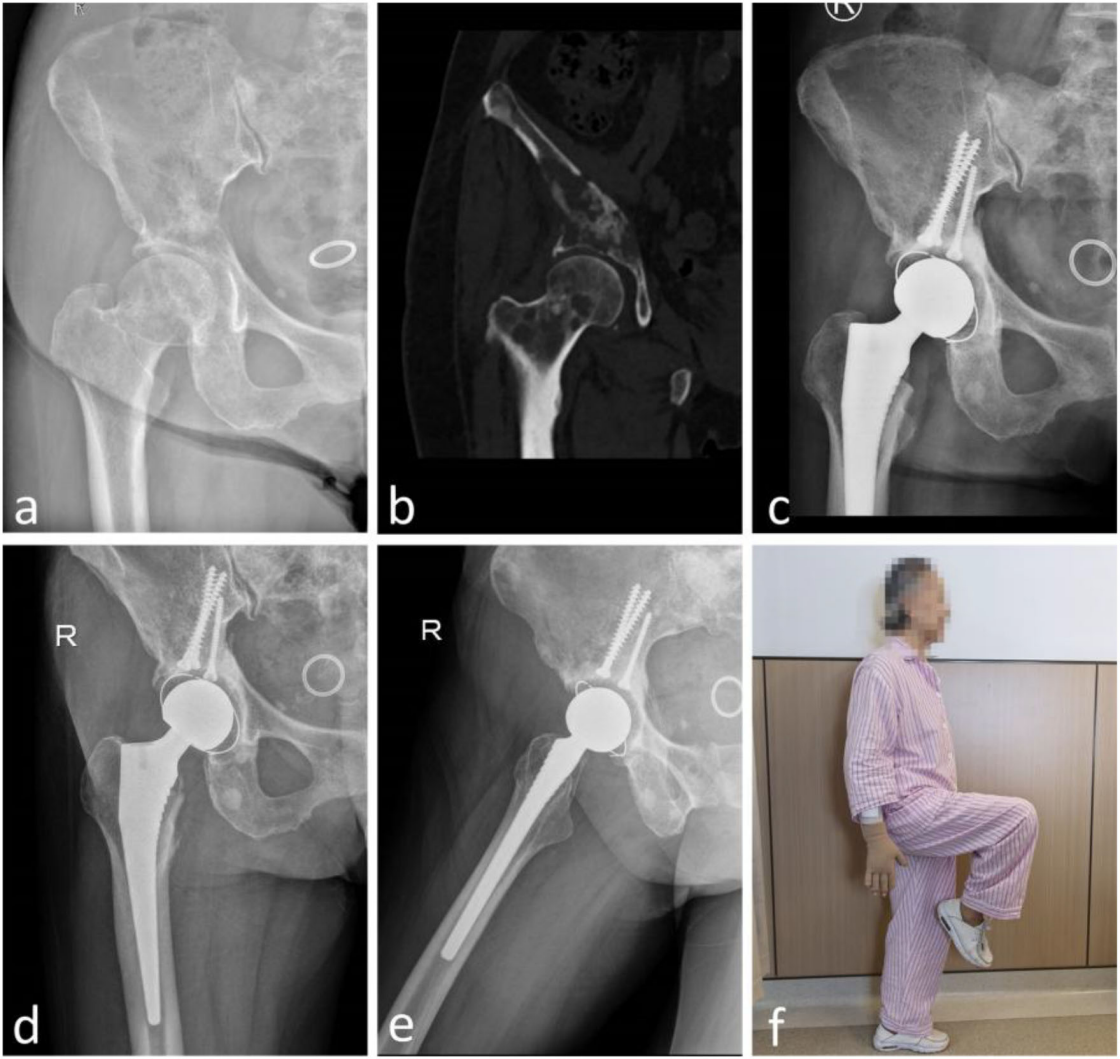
Patient 3 with breast cancer metastasis to the acetabulum treated with Screw-and-Cement Pile reconstruction using a standard polyethylene cemented cup and total hip arthroplasty. Intraoperative microwave ablation was applied to the tumor-involved region preserved at the acetabular dome. (a) Preoperative anteroposterior (AP) pelvic radiograph and (b) preoperative computed tomography (CT) showing marked lytic destruction of the acetabular roof with involvement of the femoral head and neck; (c) AP hip radiograph at 5 months demonstrating well-positioned components without screw back-out, cup migration, or loosening; (d) AP and (e) lateral hip radiographs at 16 months showing maintained component position without loosening or migration; (f) Standing lateral view at 16 months demonstrating good hip flexion.
